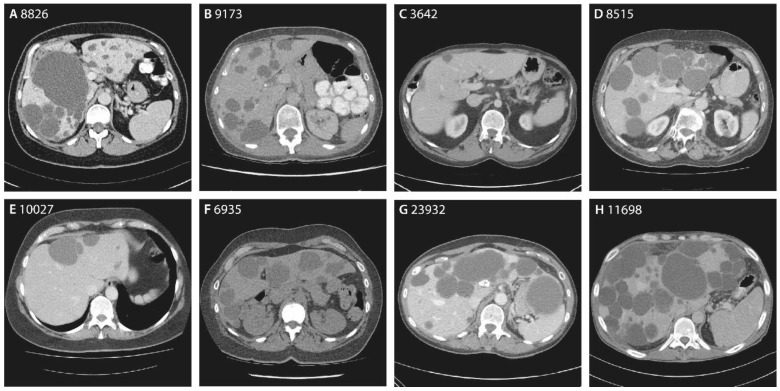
CT scans of eight ADPLD individuals with heterozygous pathogenic *ALG8* variants. (A) Individuals 8826 and (B) 9173 are part of family 1, and are carriers of the same nonsense variant (c.160C>T p.(Gln54*)). (C) Individuals 3642 and (D) 8515 are carriers of frameshift variants (c.272delA p.(Asn91Metfs*5) and c.371delG p.(Cys124Serfs*33)), (E) 10027 is a carrier of a splice-site variant (c.478+3A>G p.?), and (F) 6935, (G) 23932 and (H) 11698 are carriers of different nonsense variants (c.685C>T p.(Arg229*), c.1090C>T p.(Arg364*), and c.1501delG p.(Val501*)).
